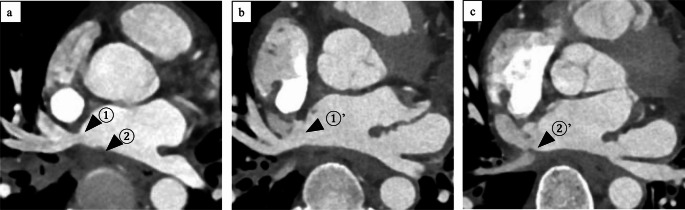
Example showing pulmonary vein stenosis before and after surgery. a Preoperative CT shows severe stenosis of the RMPV (black arrowhead ①) and obstruction of the RIPV (black arrowhead ②). b, c Postoperative CT shows dilation of the RMPV (black arrowhead ①’) and patency of the RIPV (black arrowhead ②’). CT: computed tomography, RIPV: right inferior pulmonary vein, RMPV: Right middle pulmonary vein
